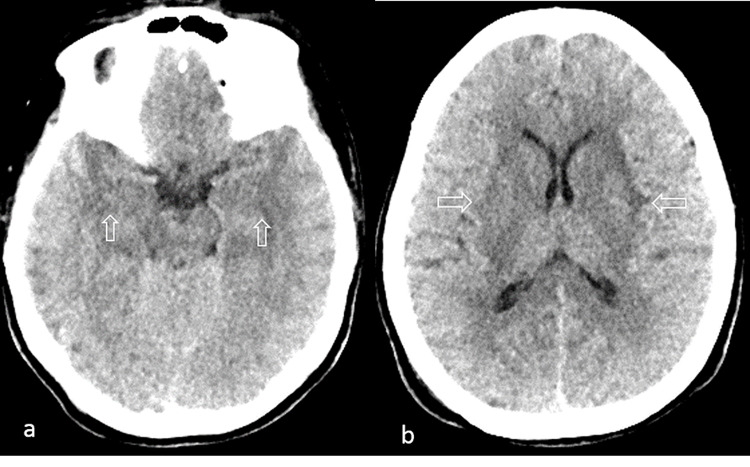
Non-contrast CT scan of the brain. Initial plain CT scan of the brain with axial cuts showing (a) bilateral cerebral temporal and fronto-parietal deep white matter periventricular hypodensities as well as (b) basal ganglia hypodensities (hollow arrows).
